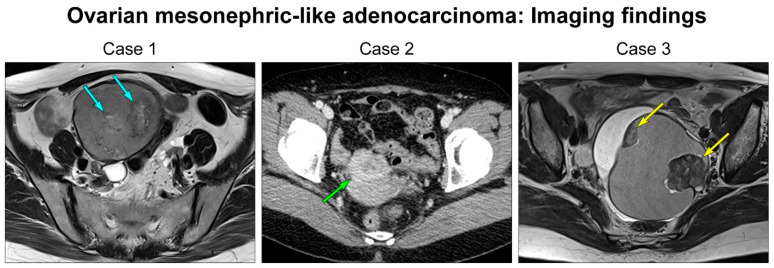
Imaging and gross findings of ovarian MLA. Pelvic magnetic resonance imaging (cases 1 and 3) and computed tomography (case 2) revealed cystic ovarian masses containing internal solid nodules (blue, green, and yellow arrows).
